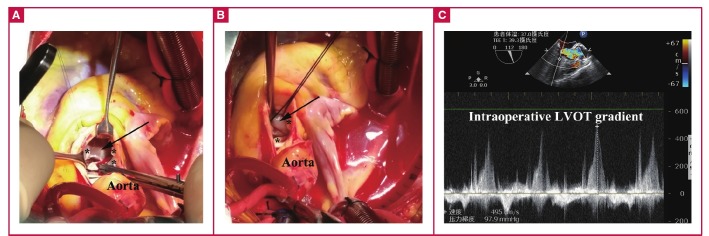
The sharp angle of the basal IVS (A, black arrow) and anomalous long PM (B, black arrow) were demonstrated through a transaortic approach (* aortic valve). After the first recovery of heart beat, transoesophageal echocardiography showed the LVOT gradient was still about 100 mmHg (C).
